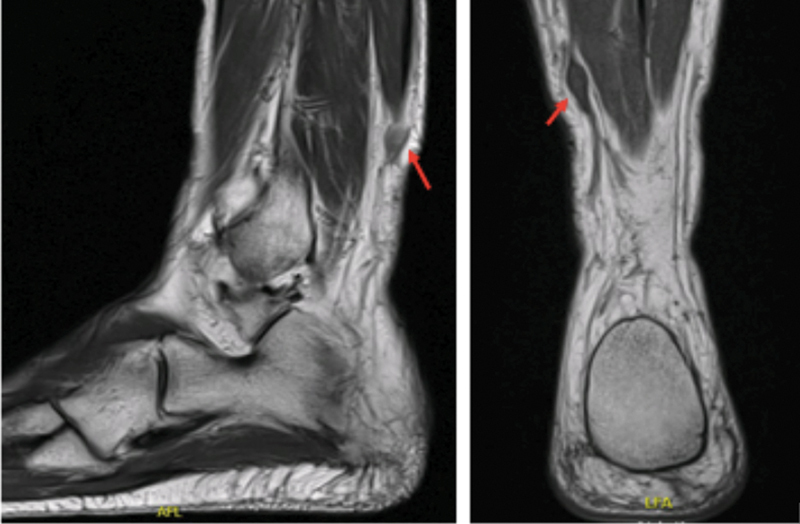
Magnetic resonance imaging (MRI) scan revealed hypointensity on T1.
